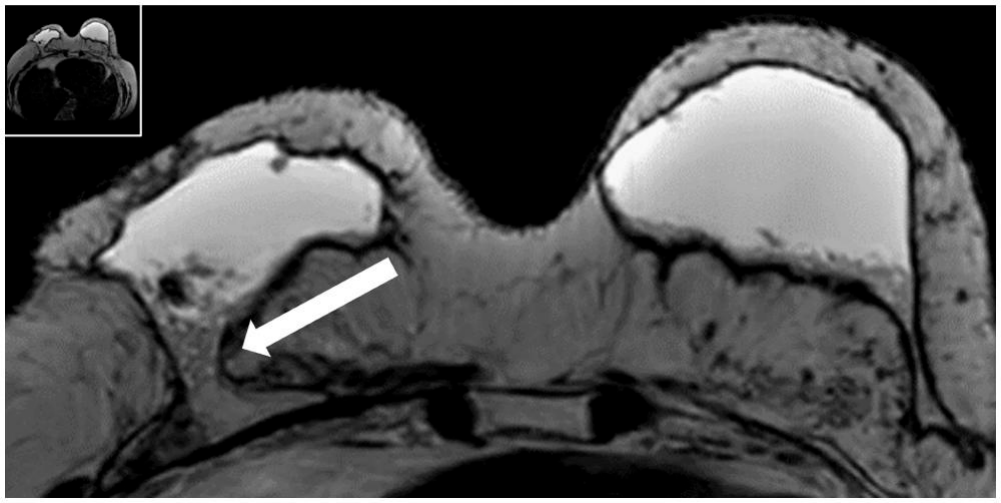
Breast seroma after total breast reconstruction with AFT on MRI. This is a breast MRI of the same 53-year-old patient that had a persistent seroma despite multiple drainage treatments and sclerotherapy. The axial T2-image shows bilateral hyperintense collections. The white arrow demonstrates that the right fluid collection is continuous with the pectoral muscle and a gravity dependent gradient/layering of fluid-debris is seen. Abbreviations: AFT = autologous fat transfer; MRI = magnetic resonance imaging.
